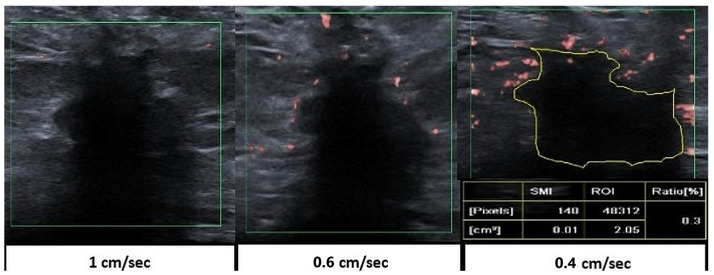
Superb Microvascular Imaging (SMI) assessment of the post-neoadjuvant chemotherapy (NAC) hypoechoic tumor bed. Perilesional flash artifacts are demonstrated at reduced velocity scale settings to enable detection of very-low-velocity flow signals within the hypoechoic tumor bed. During bedside ultrasound response evaluation, the SMI index value was obtained using a free-hand region-of-interest (ROI) technique, in which the borders of the hypoechoic tumor bed were manually delineated to achieve optimal boundary definition. In this study, measurements were taken within the velocity scale range of 0.4–0.6.
